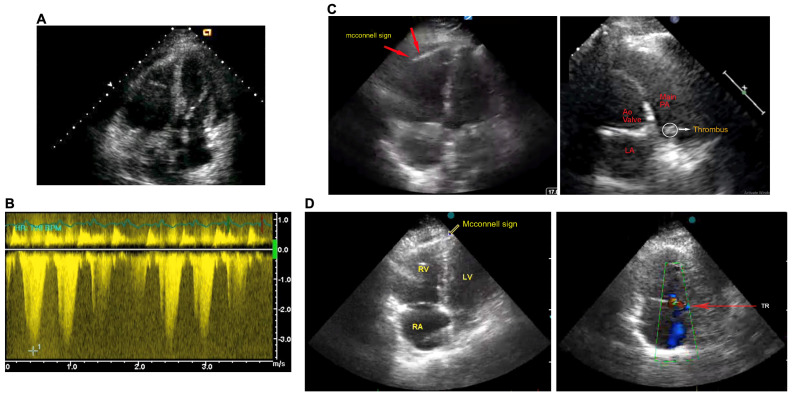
The transthoracic echocardiogram analysis in Russell’s viper bite patients. The echocardiogram was performed on patients 1 (A,B), 2 (C), and 3 (D). (B) shows pressure in the pulmonary artery. The typical McConnell’s sign is shown in relevant images. RV—right ventricle; LV—left ventricle; RA—right atrium; AO—aortic valve; PA—pulmonary artery; LA—left atrium; TR—thrombus. The examination was carried out on the day of admission at Manian Medical Centre.
